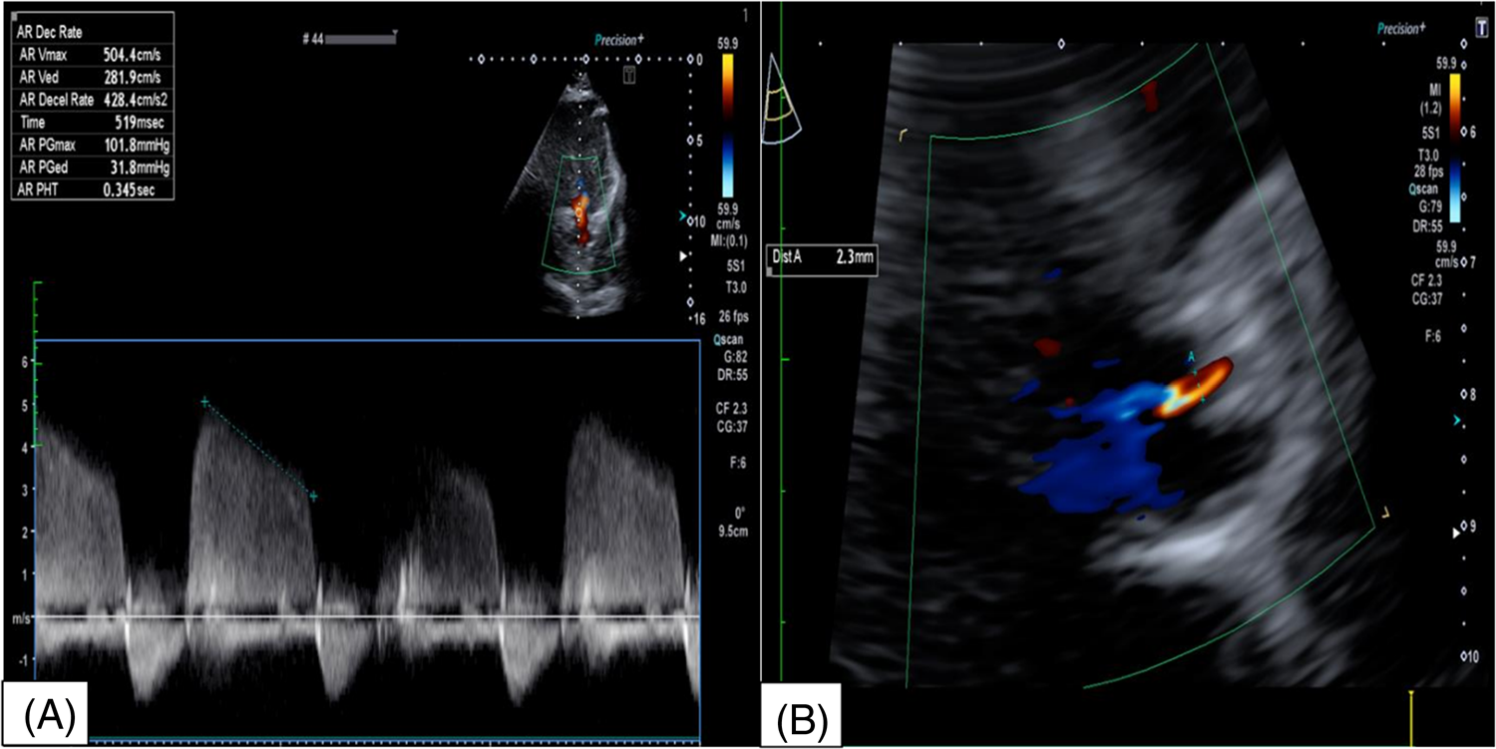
(A) Pre operative. Quantification of QAV regurgitation with pressure half‐time. (B) Follow up transthoracic echocardiogram, quantification of residual aortic valve regurgitation with vena contracta width after QAV repair.
